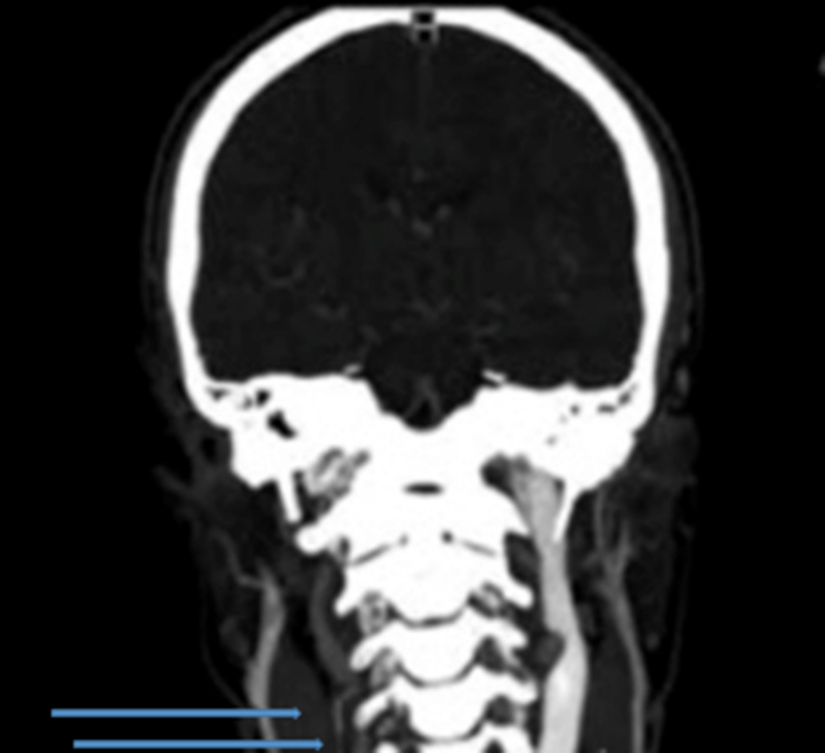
Magnetic resonance imaging with evidence of internal jugular agenesis.
